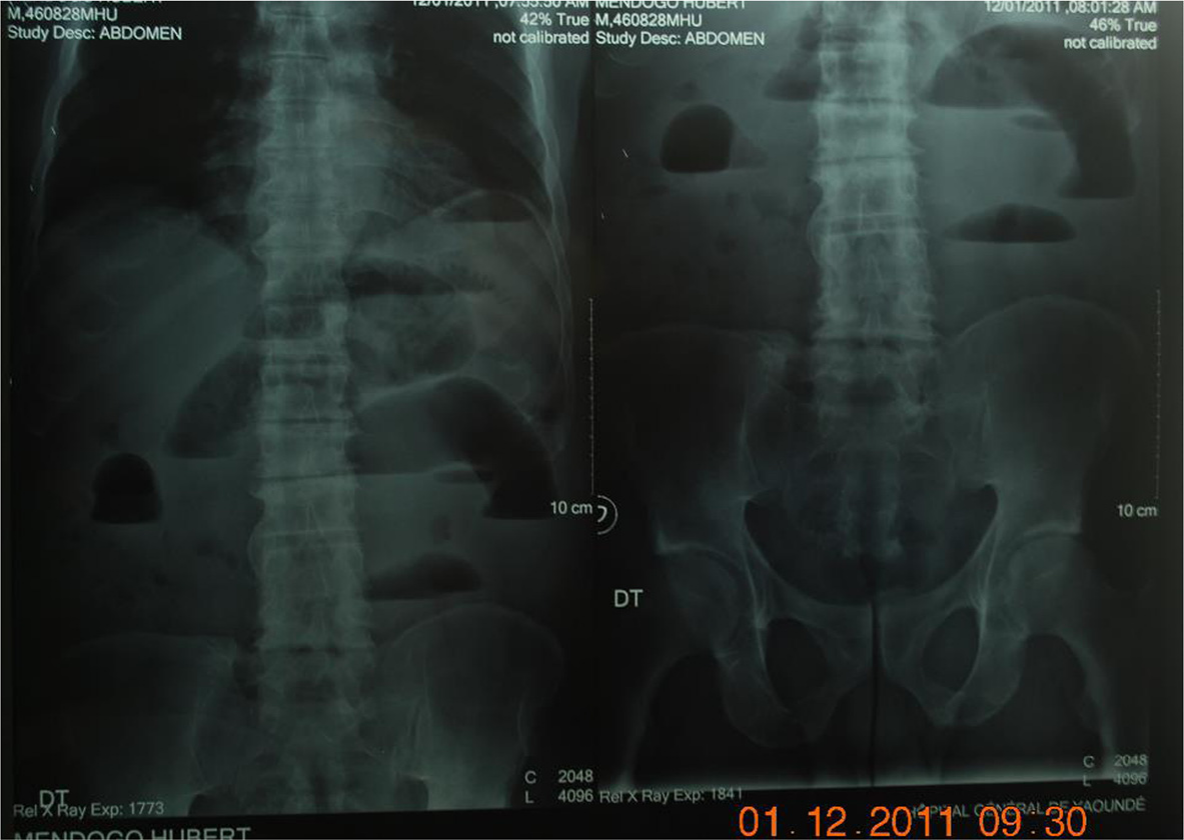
Plain upright abdominal X-ray, taken preoperatively demonstrates Gas shadow in the anabdomen.
